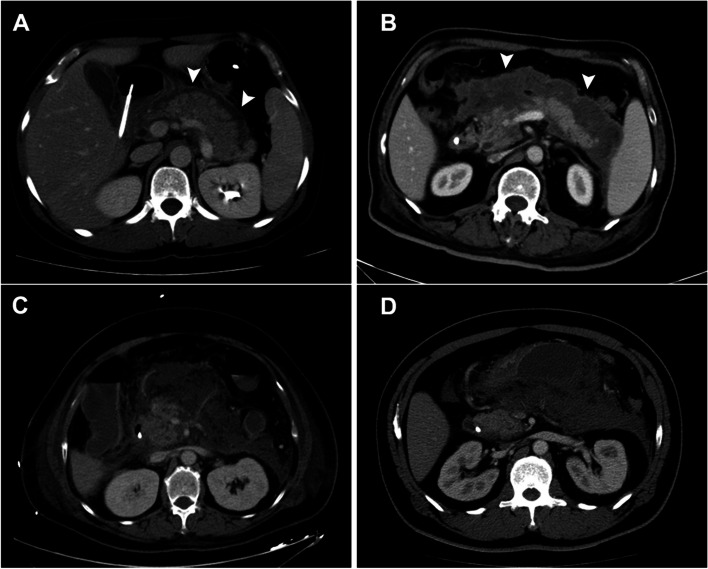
Axial contrast-enhanced CT scans in acute pancreatitis patients. A, B with encapsulation; C, D: without encapsulation. A 52-year-old woman on day 5 after symptom onset; (B) 71-year-old man on day 9 after symptom onset; (C) 59-year-old woman on day 8 after symptom onset; (D) 49-year-old man on day 9 after symptom onset. Arrowheads denote the encapsulation around the peripancreatic fluid/necrosis collections
